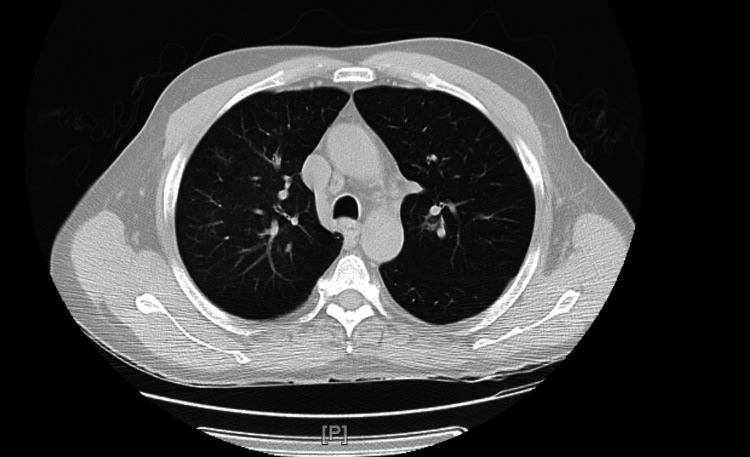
CT scan eight months after steroid therapy showed complete disappearance of the lung mass.
